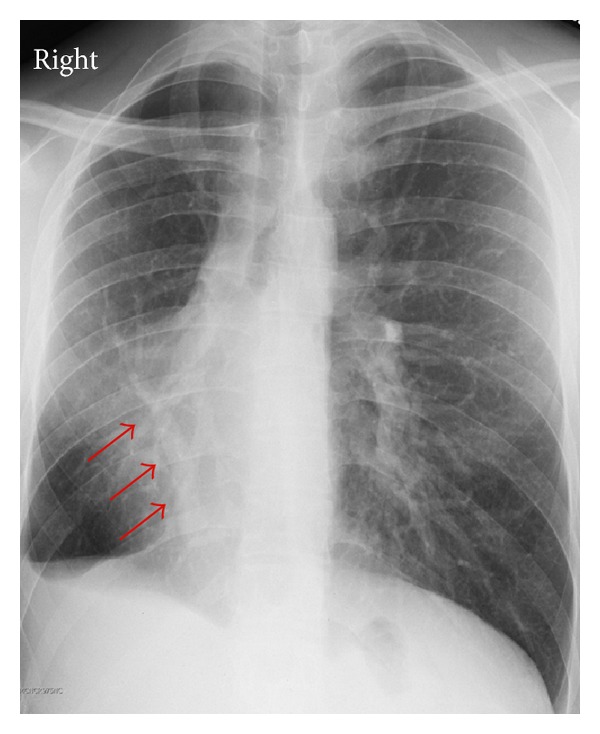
Chest radiograph shows decreased space associated with the hypoplastic lung and displacement of the cardiac silhouette to the right (scimitar sign) (arrows).
